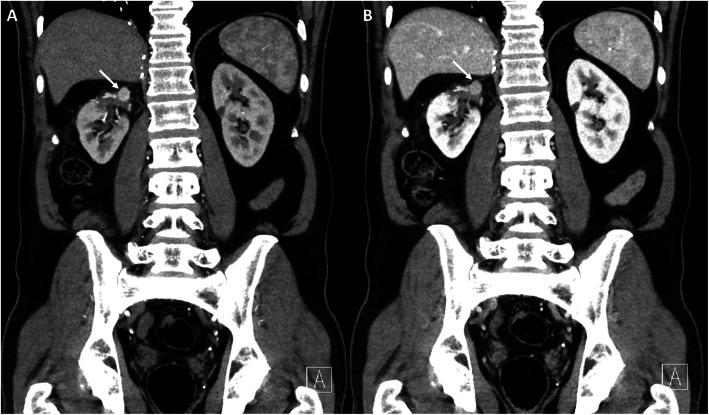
Coronal CT images of the local recurrence in arterial (A) and portal venous (B) phases of a 64-year-old man with multiple metastatic RCC
